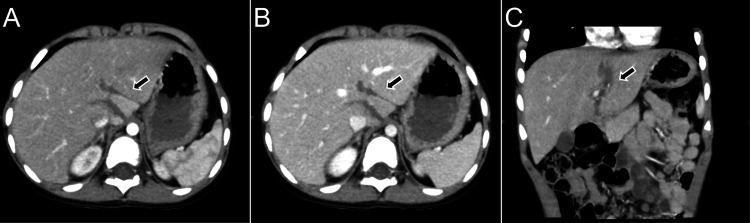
Initial contrast-enhanced computed tomography of the abdomen The initial CECT performed on the day of injury showing grade III laceration of segments V and I of the liver, representing deep hepatic injury with no extravasation (black arrow). (A) Axial view of CECT in the late arterial phase, (B) axial view of CECT in the portal phase, and (C) coronal view of CECT in the late arterial phase.
